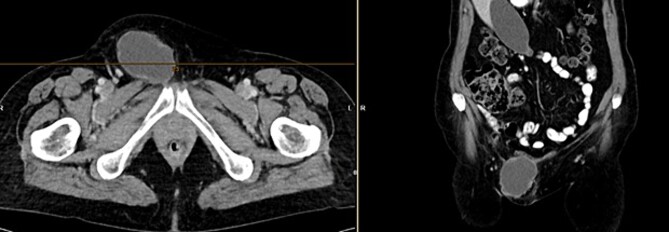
CT of the abdomen from 25 February 2022: oval encapsulated structure in the right groin area, most likely soft tissue hematoma, no active bleeding, DD enlarged lymph node.
